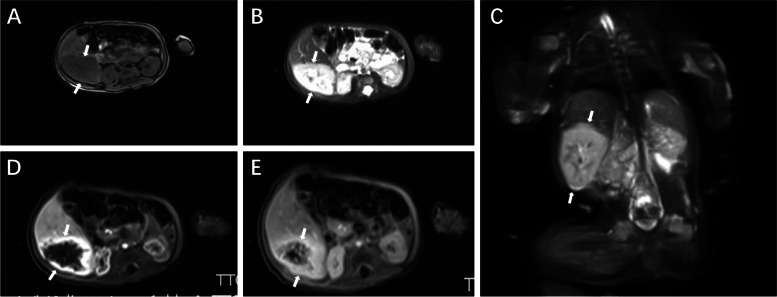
Postnatal MRI showed a large mass in the right lobe of the liver. A-C The transverse and coronal views of MRI showed a large mass in the right lobe of the liver, approximately 6.0 × 4.1 cm in size, low T1-W and high T2-W signals without signs of a false envelope, and adjacent compression of the right kidney. D-E Uneven enhancement of the edge of the mass was observed in the arterial phase of enhanced scanning. With the delay in scanning time, the enhancement area gradually widened and expanded to the center, but was not completely filled
